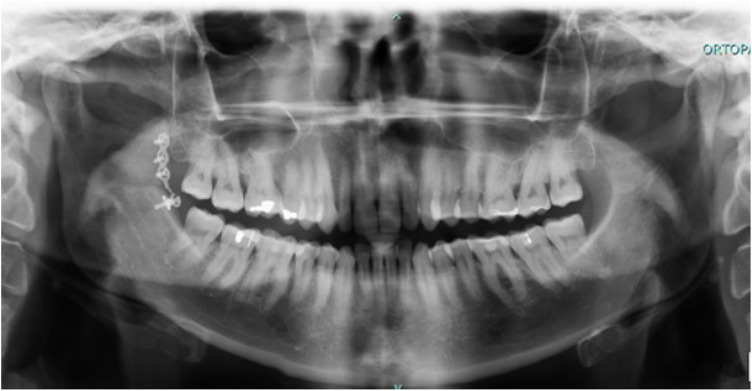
Postoperative orthopantomography (6 months after surgery).
